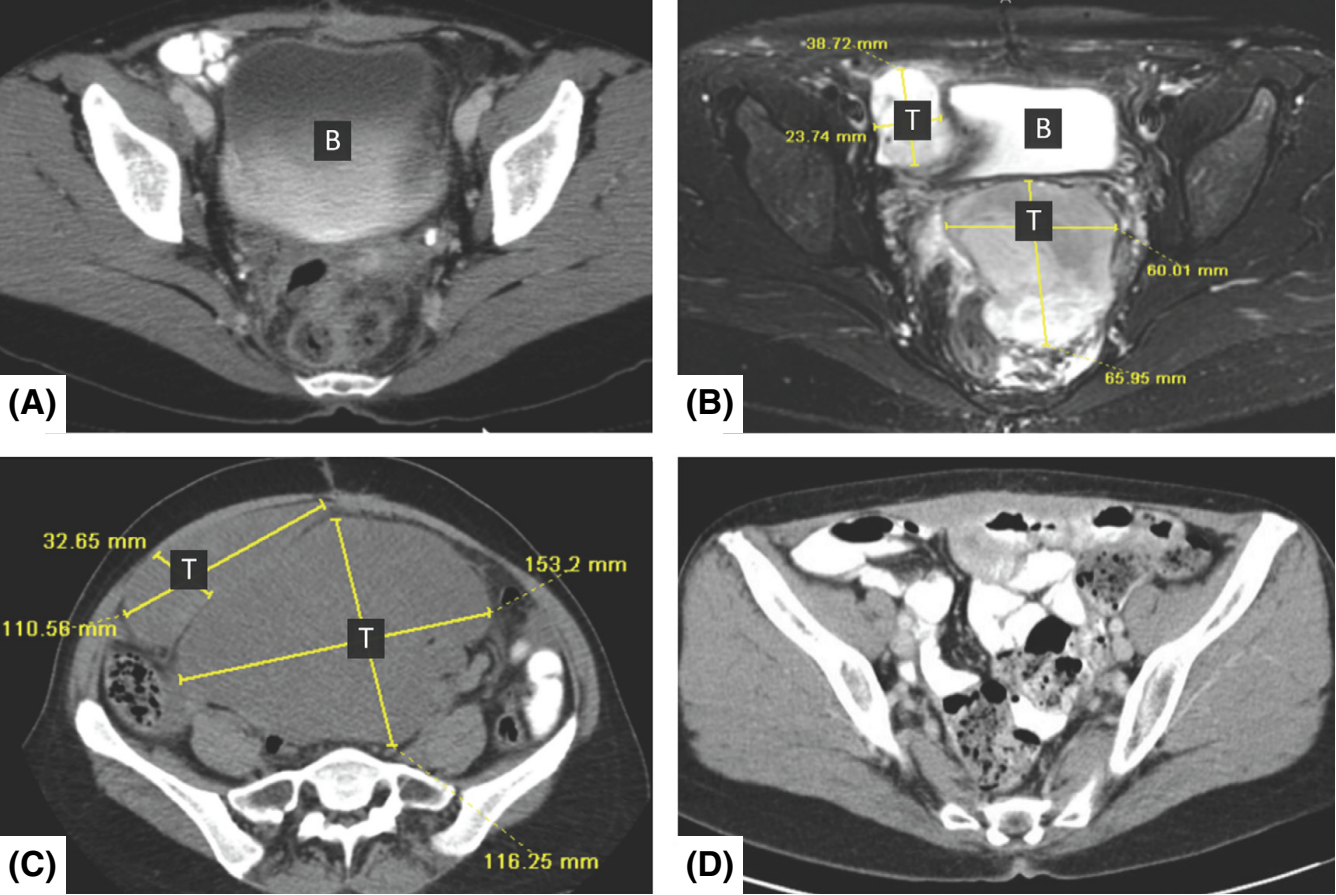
Rapid tumor recurrence. (A) Computed tomography (CT) scan of the pelvis 1 month after dubulking of pelvic recurrence and 8 months following TAH/BSO. No evidence of disease. (B) Magnetic resonance imaging (MRI) of the pelvis 1 month after image A showing two pelvic masses (65 × 60 mm and 23 × 38 mm). (C) CT scan of the pelvis 1 day before CRS/HIPEC showing rapidly growing tumors (116 × 153 mm and 110 × 32 mm) 4 weeks after MRI presented in image B. (D) CT scan of the pelvis, 5 years after CRS/HIPEC showing no evidence of disease recurrence. B, bladder; T, tumor; CRS/HIPEC, cytoreductive surgery and hyperthermic intraperitoneal chemotherapy.
